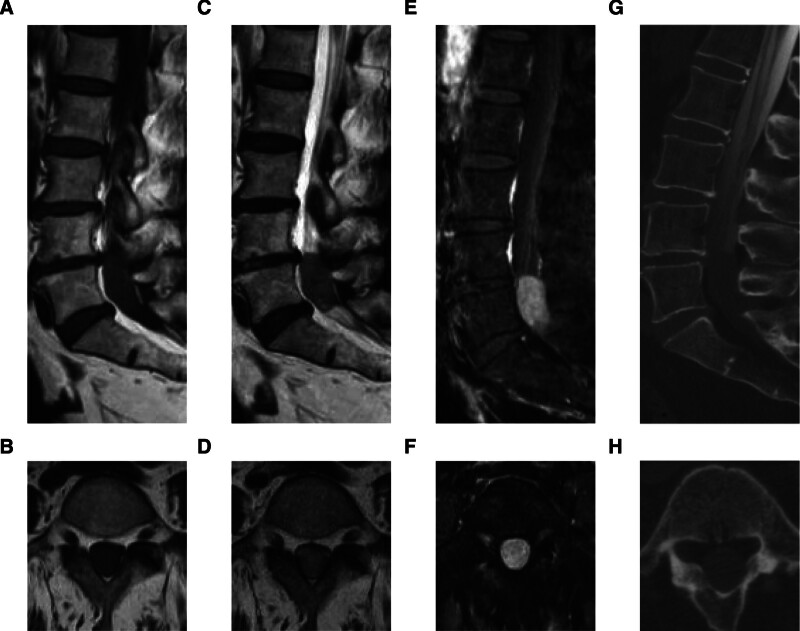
Imaging findings of clear cell meningioma (CCM). (A and B) Preoperative T1-weighted magnetic resonance imaging (MRI) in the sagittal (A) and axial (B) sections. (C and D) Preoperative T2-weighted MRI in the sagittal (C) and axial (D) sections. (E and F) Preoperative gadolinium-enhanced T1-weighted MRI in the sagittal (E) and axial (F) sections. (G and H) Computed tomography (CT) images following myelography in the sagittal (G) and axial (H) sections.
